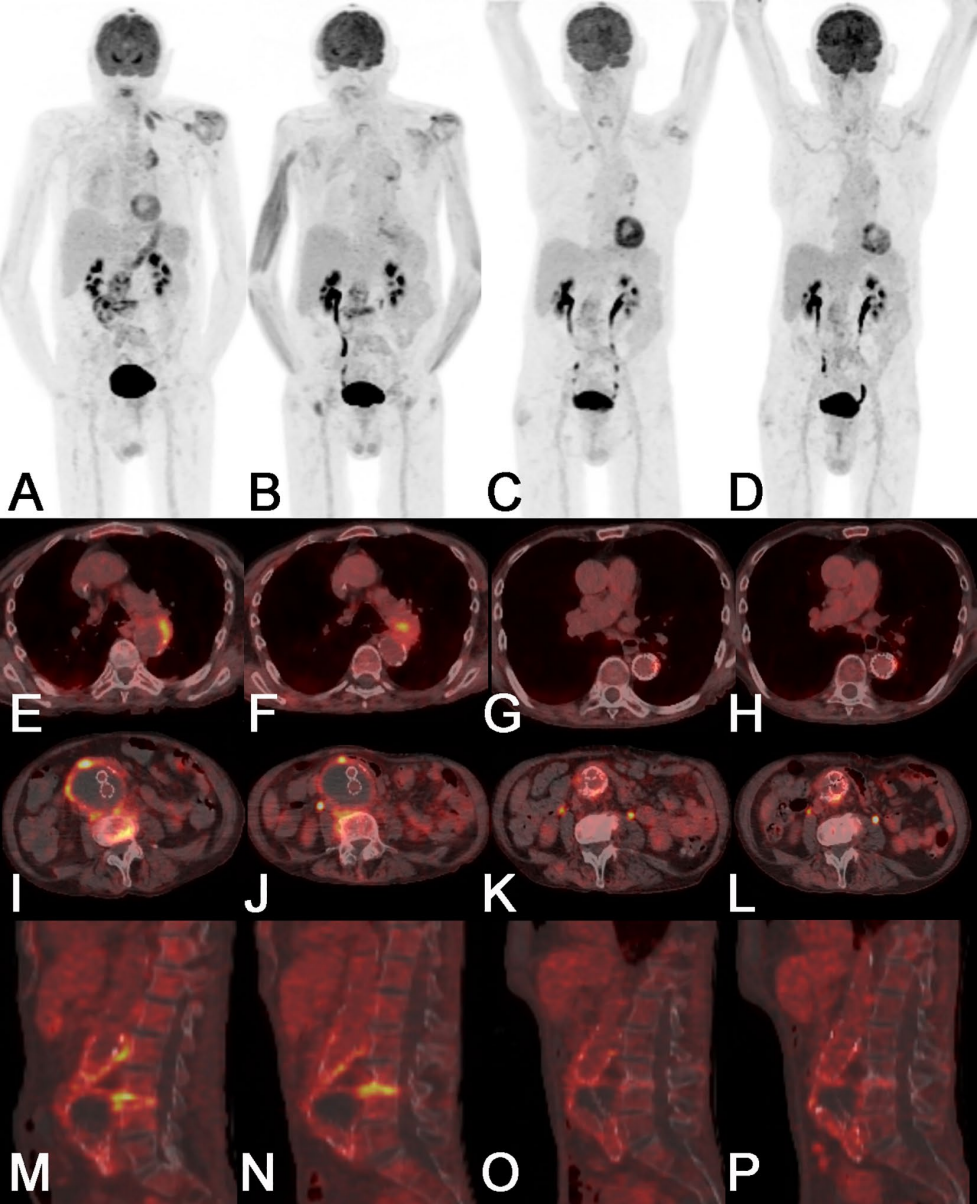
PET/CT of an 82-year old male patient (patient 05 in Tables 1, 2 and 3) with a vascular graft infection and spondylodiscitis due to *Streptococcus agalactiae* showed a new focal FDG uptake in the wall of the thoracic aorta in September 2017. Both readers rated the thoracic finding as a infective native aneurysm despite the fact that the vessel diameter was not pathologically widened. The first PET/CT follow-up in revealed a progression of the aneurysm in size with stable increased FDG uptake; at the same time the FDG uptake of the spondylodiscitis increased while it partially decreased in the vascular graft infection; C-reactive protein and white blood cell count decreased. After subsequent thoracic endovascular repair with an Endurant II Stent Graft Systems (MEDTRONIC), two further PET/CT follow-up, before and after termination of antimicrobial therapy (223 days of therapy) showed faint residual FDG uptake in all sites of infection. At the last clinical follow-up in January 2019 the patient was in good clinical condition with no sign of infection. *Note*: Panels A-D show maximum intensity reconstructions of PET; Panels E-P show fused PET/CT images. Abbreviations: PET: positron emission tomography; CT: computed tomography; FDG: 18F-fluorodeoxyglucose.
